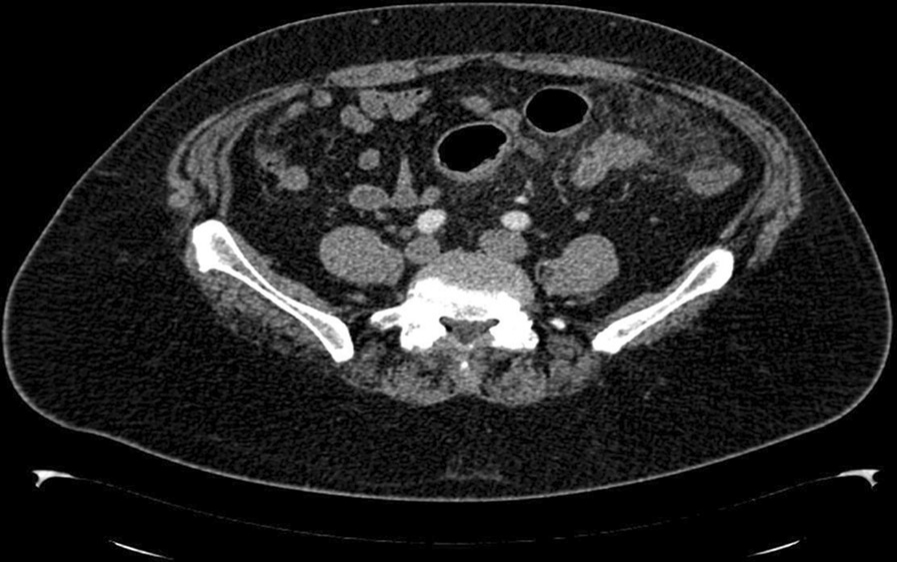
CT-scan findings of mesocolic panniculitis one year later. Axial contrast-enhanced abdominal CT scan performed one year later showed significant improvement in the radiologic appearance of the mass with regression in size and disappearance of inflammatory signs in comparison to the first study.
